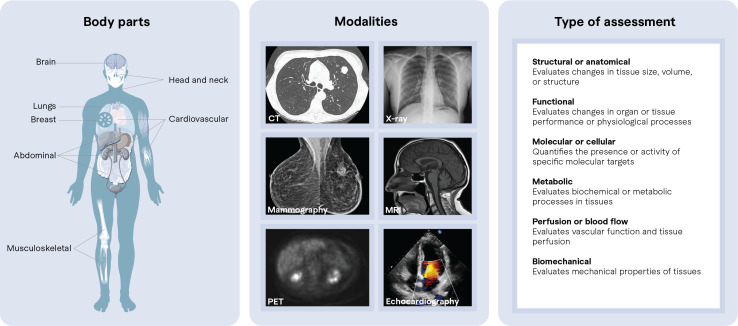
Body parts, modalities, and types of assessment for radiology images. CT: computed tomography; MRI: magnetic resonance imaging; PET: positron emission tomography.
